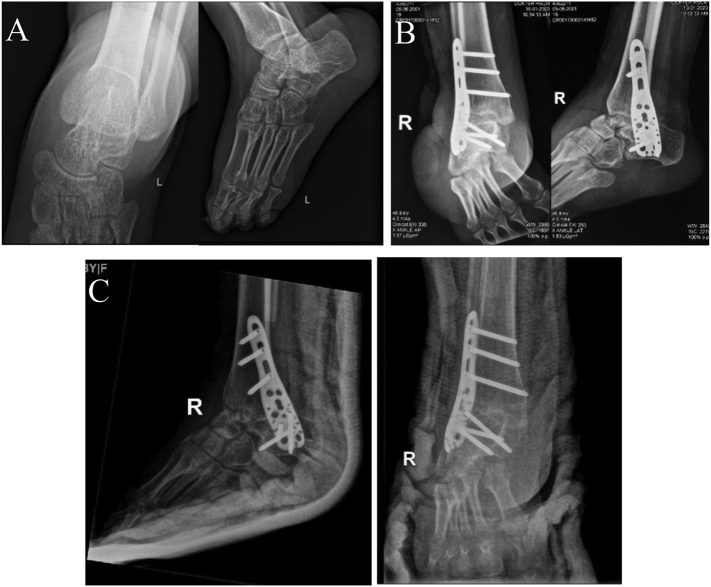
(A) Preop X-ray of the right ankle, (B) post 1st stage deformity correction using Z-plasty, total talectomy, and tibiocalcaneal arthrodesis, (C) post 2nd stage deformity correction using posteriomedial release, tendon lengthening (Tibialis posterior, FDL, FDB) and plantar fascia release.
